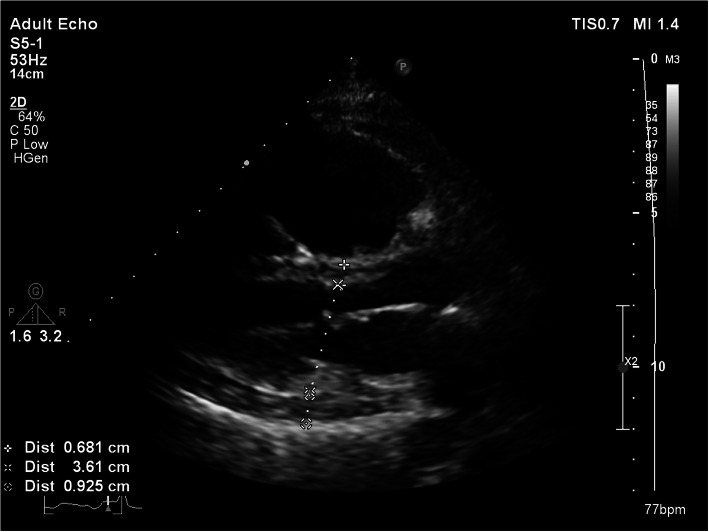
Parasternal long axis TTE view showed mild pericardial effusion in the first patient
